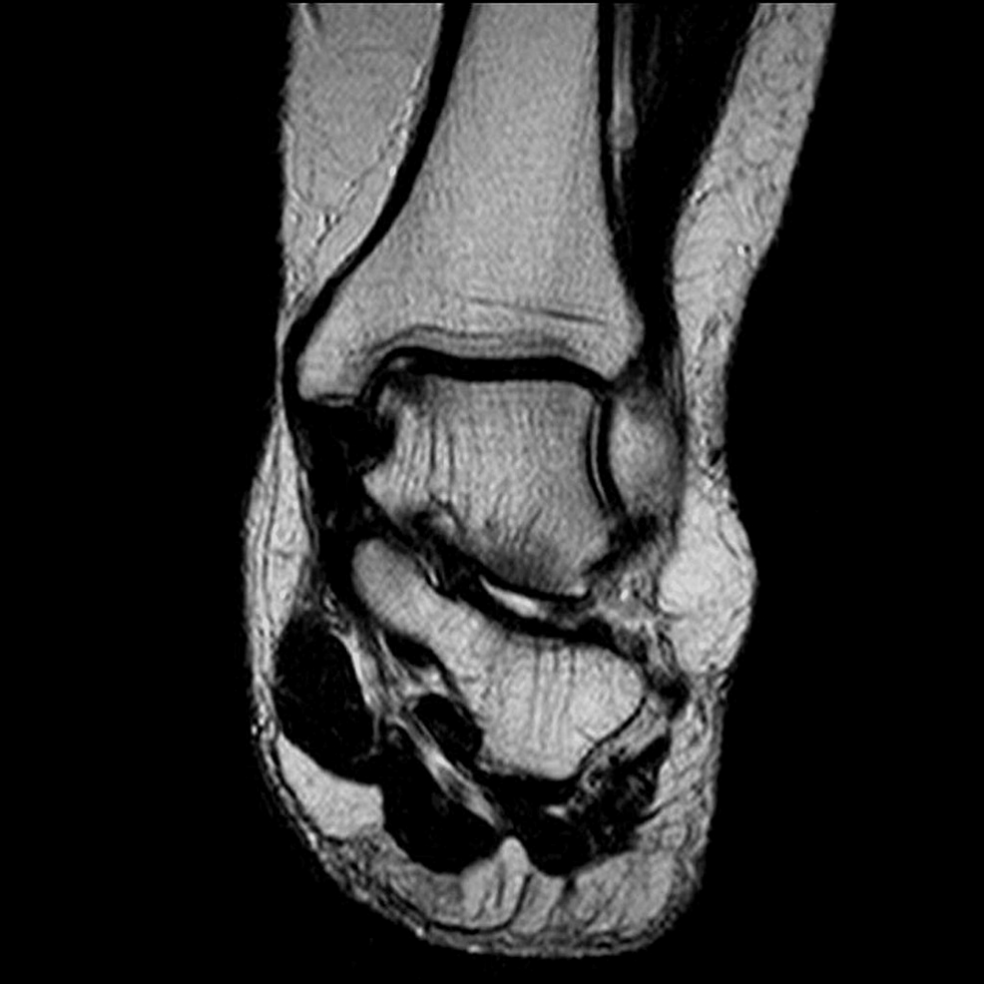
Talus medial osteochondral lesion. Preoperative MRI images: coronal section
